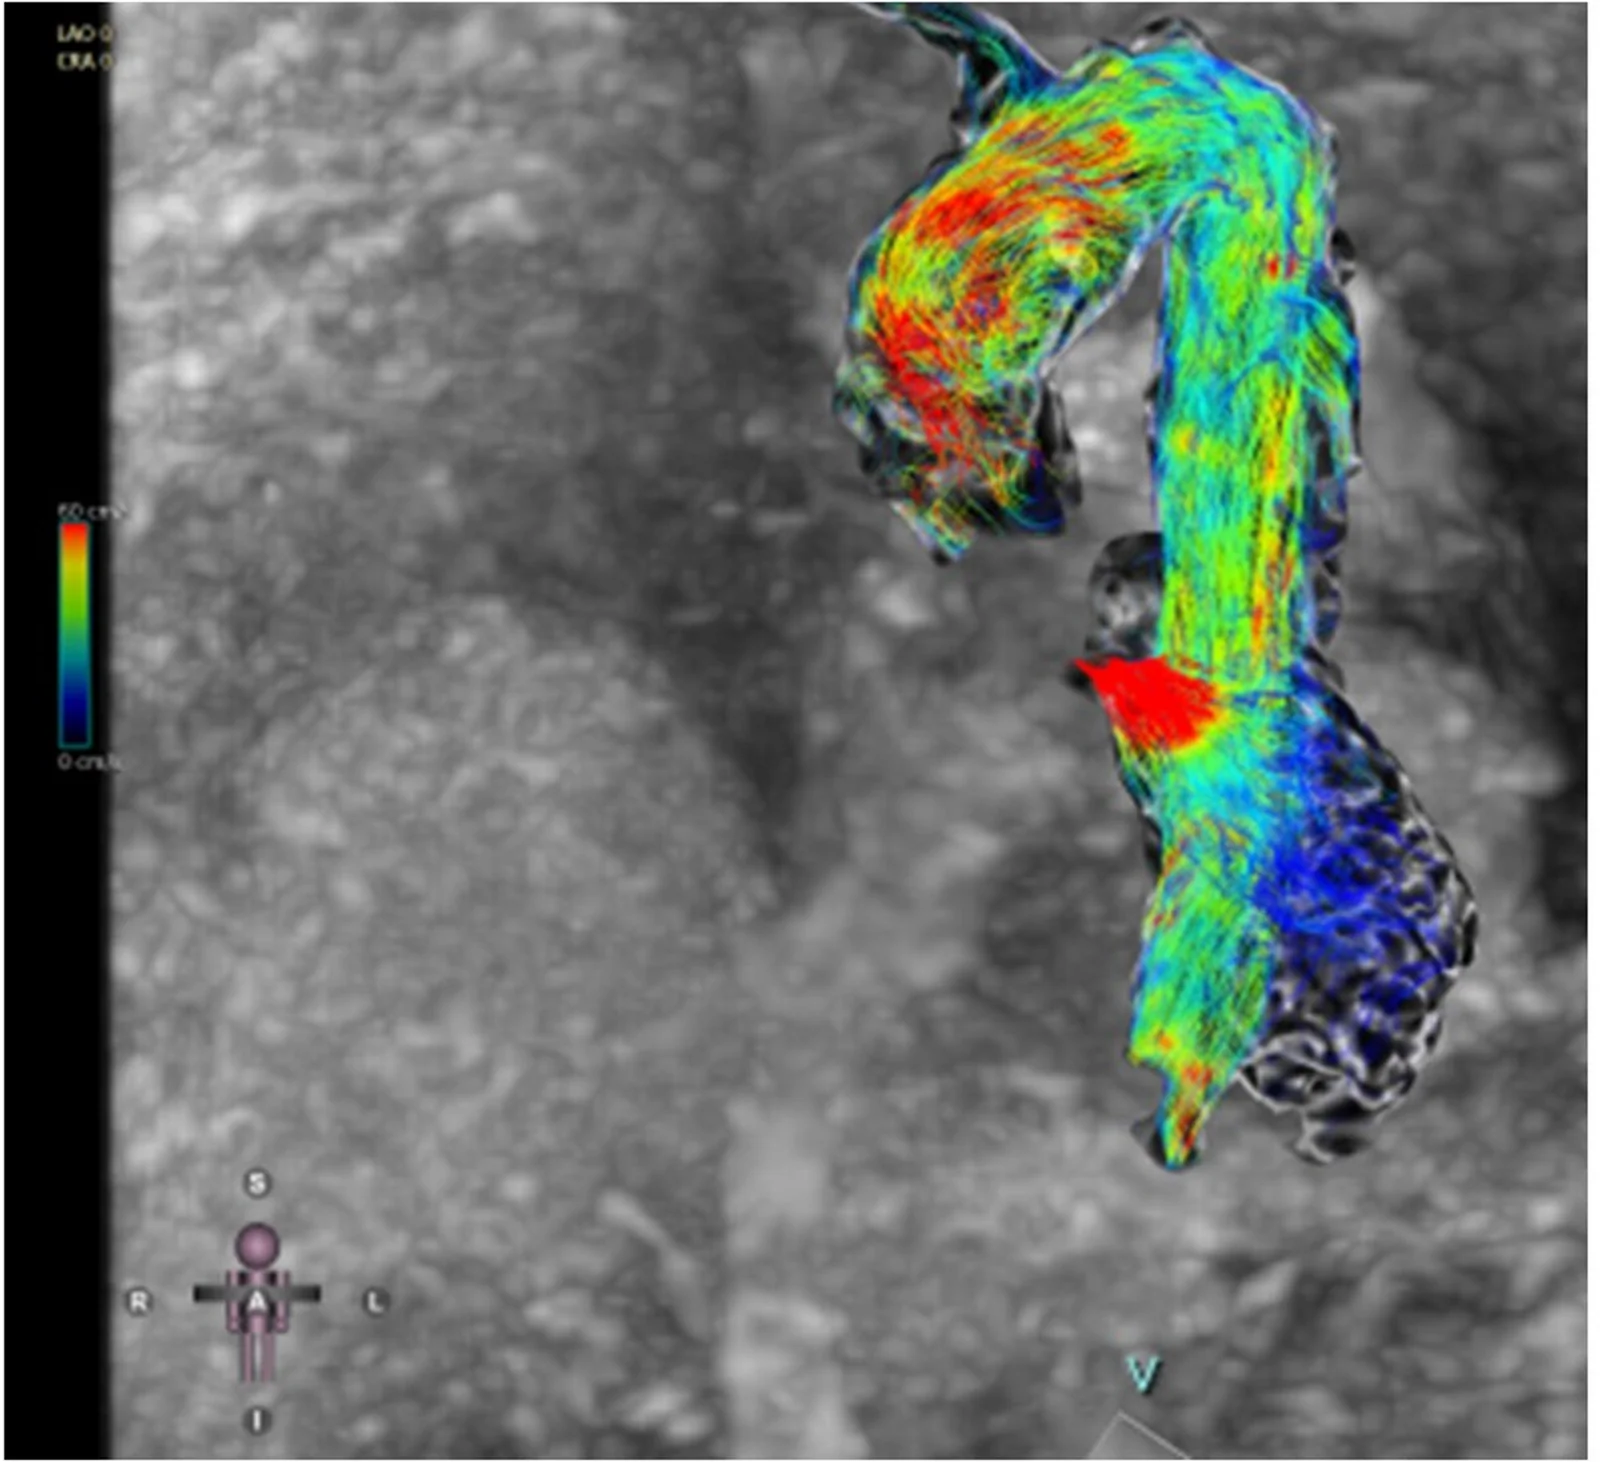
Four-dimensional flow magnetic resonance imaging. Four-dimensional flow magnetic resonance imaging (4D-flow MRI) streamline visualization showing accelerated systolic flow at the reconstructed left ventricular outflow tract (LVOT), corresponding to the narrowed segment identified on computed tomography, indicating functional LVOT stenosis
